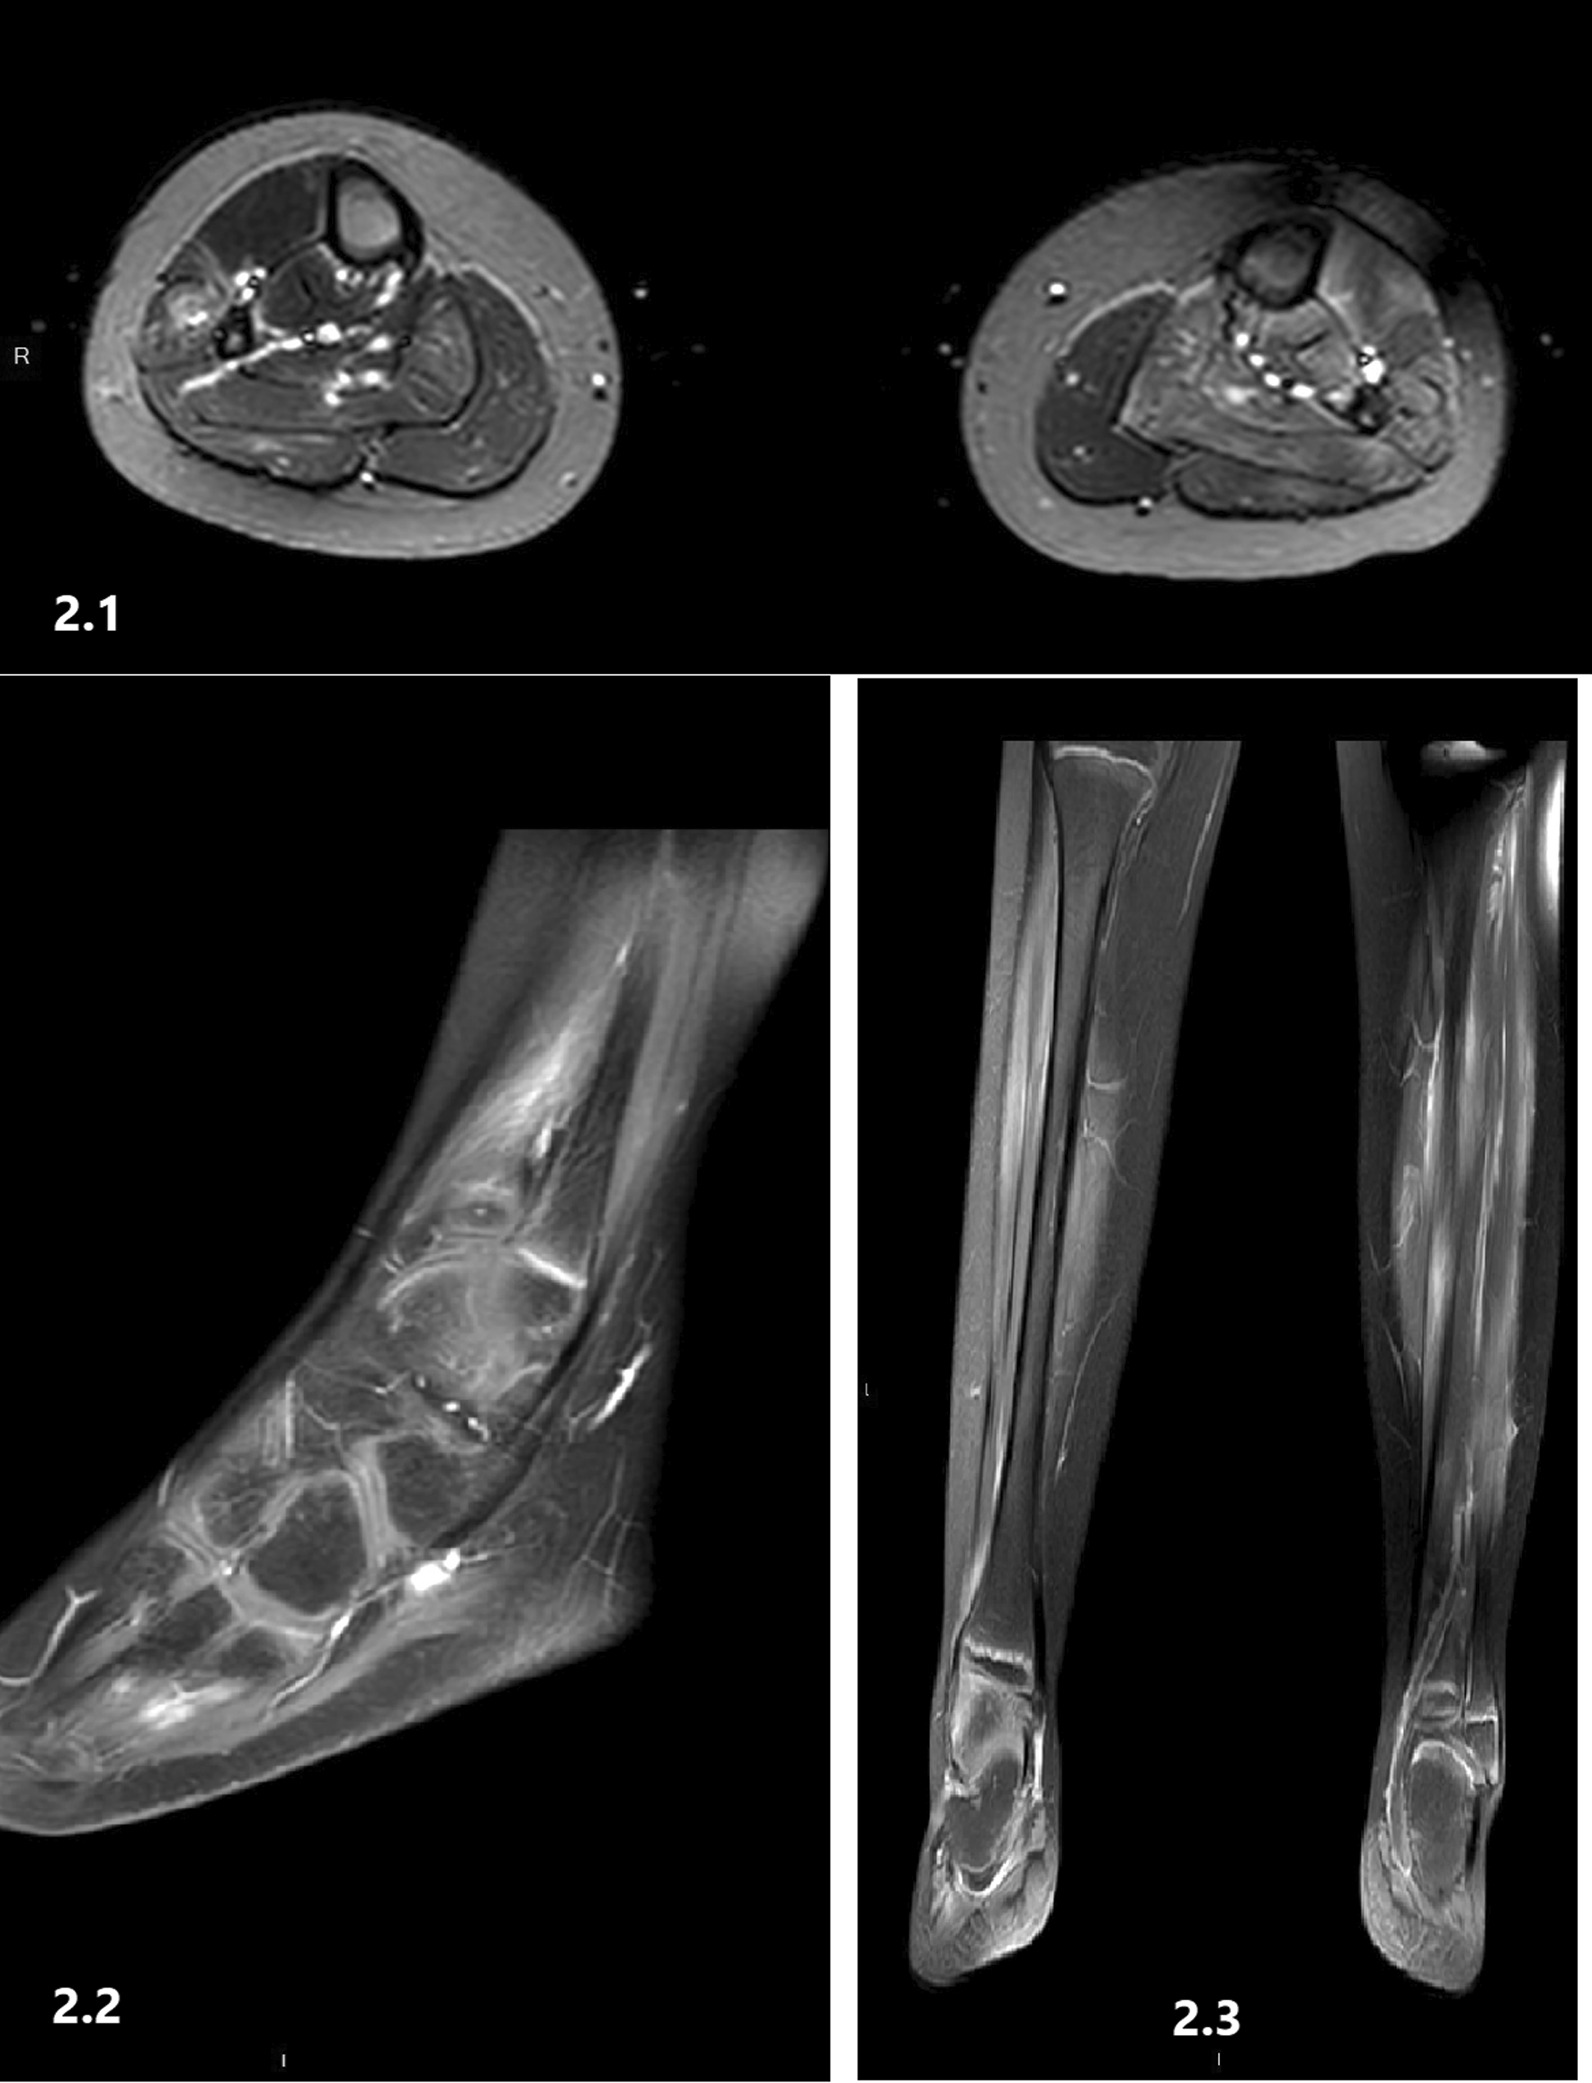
MRI of the legs (Images 2.1, 2.2, 2.3), patient number 2, shows a high signal on T2-weighted images with calf muscle enhancement after gadolinium injection compatible with myositis. The bone marrow signal is normal
